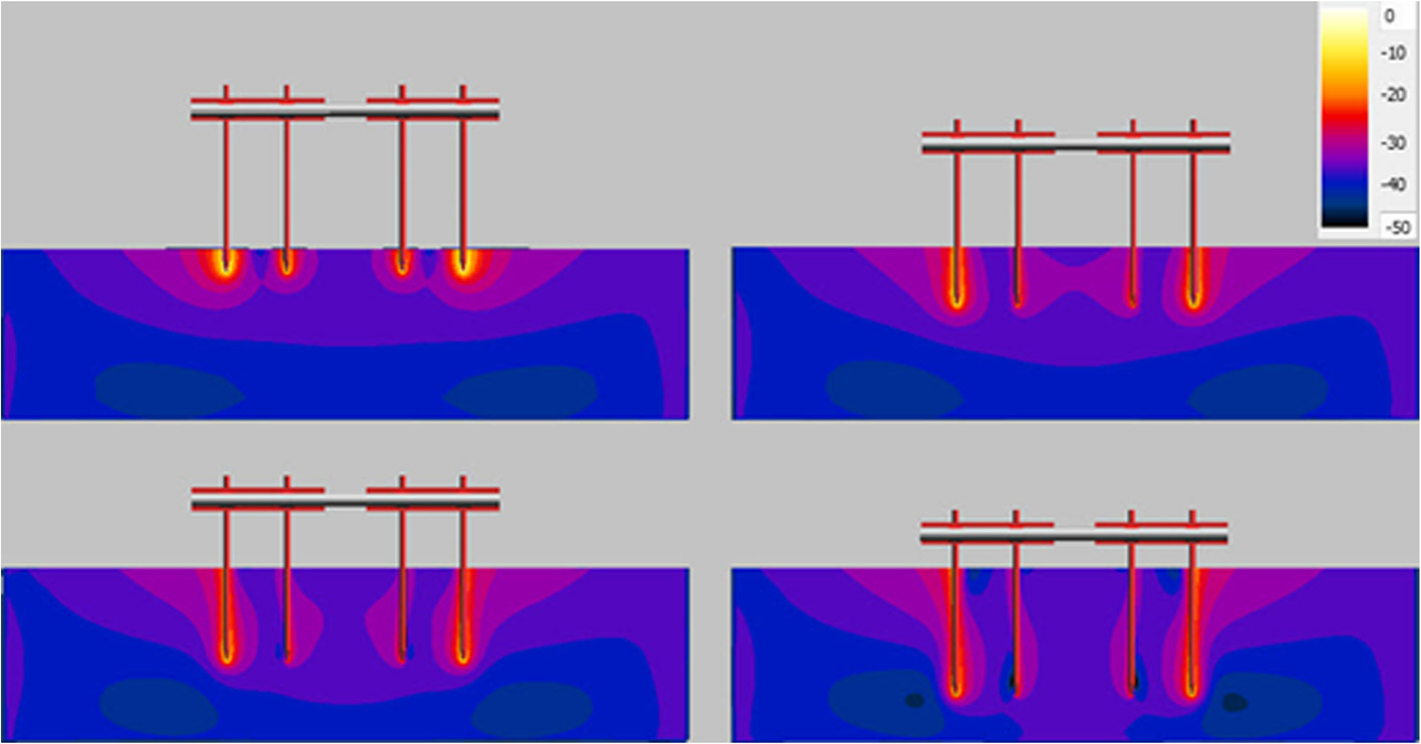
Power loss along the device pin with different insertion depth in 1.5-T MRI.
